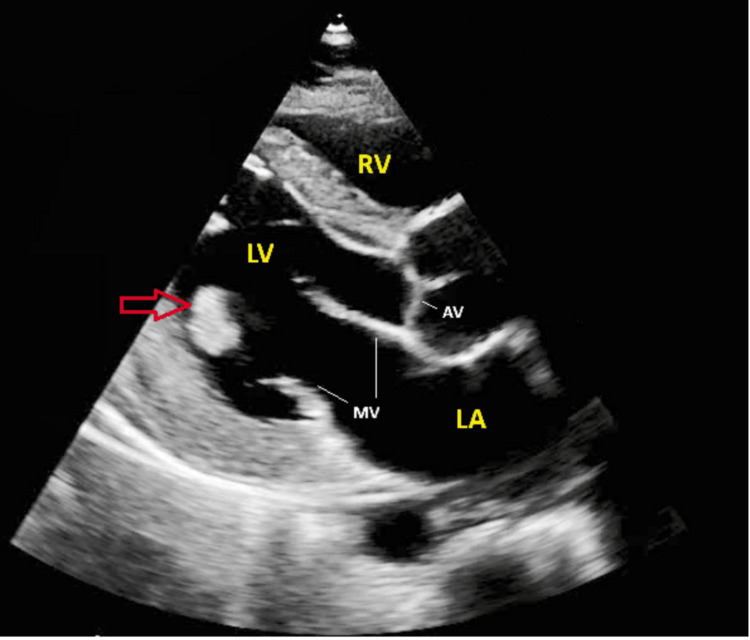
Transthoracic echocardiography The red arrow indicates a thrombus in the left ventricle (LV), attached to the papillary muscle and the posterior leaflet of the mitral valve. LV: left ventricle, RV: right ventricle, LA: left atrium, AV: aortic valve, MV: mitral valve.
